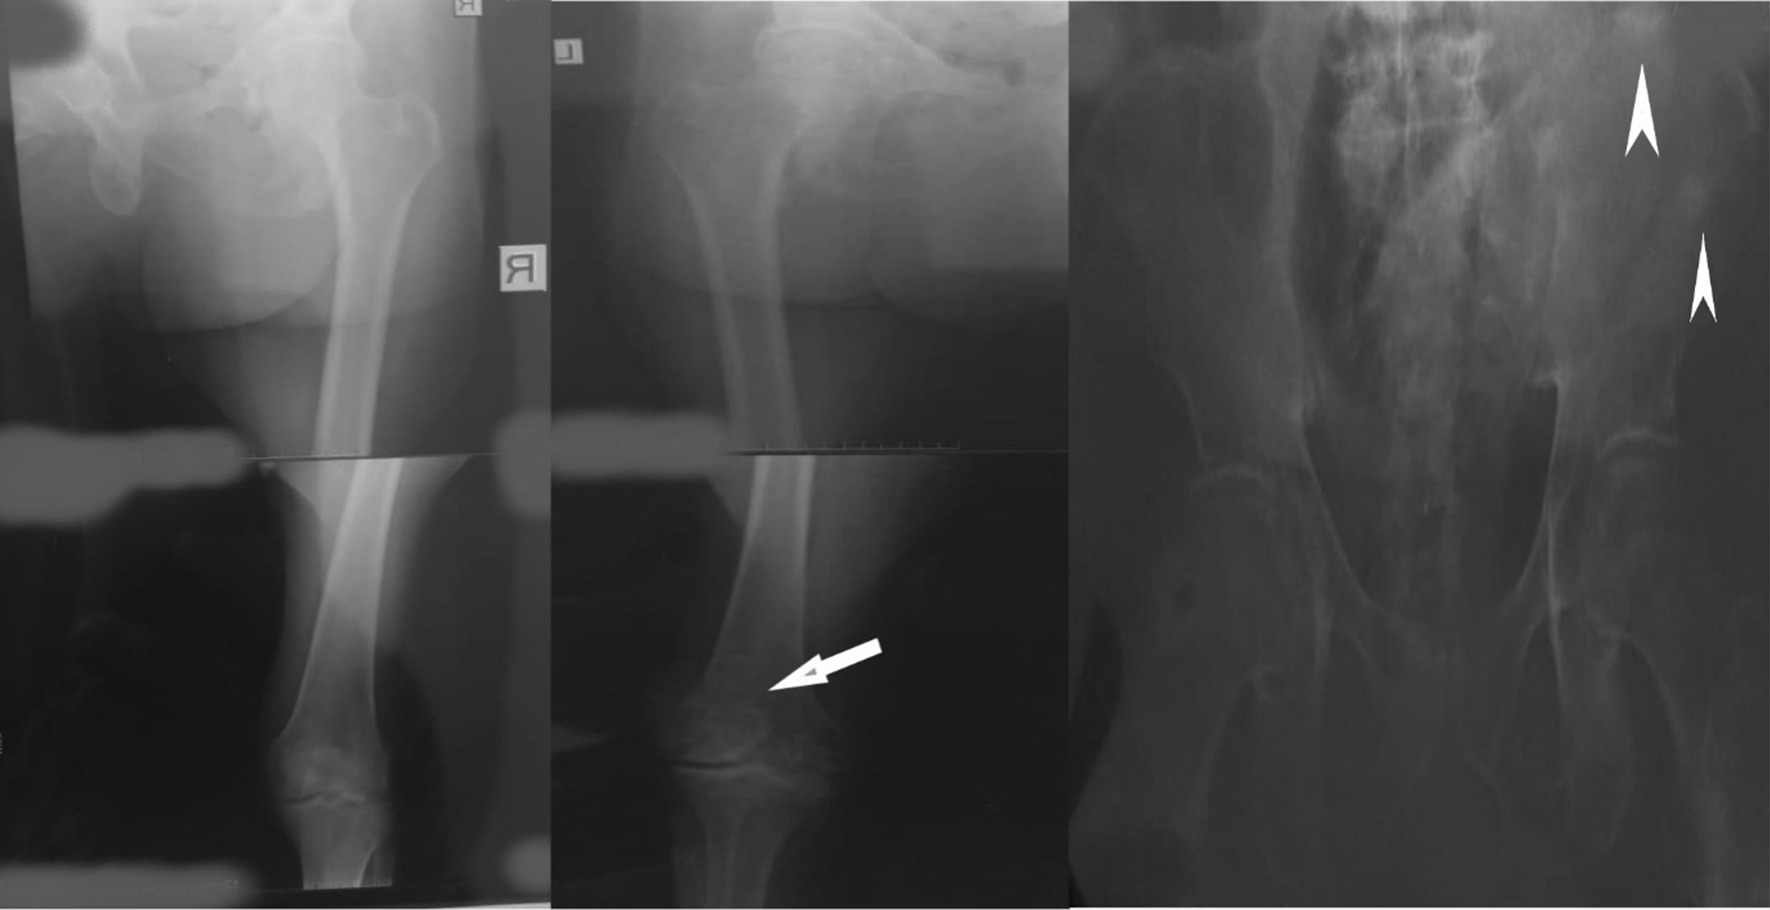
X-ray of the pelvis and right and left femur showing sclerotic lesions on the left iliac bone (arrowheads) and left lower femur (arrow)
